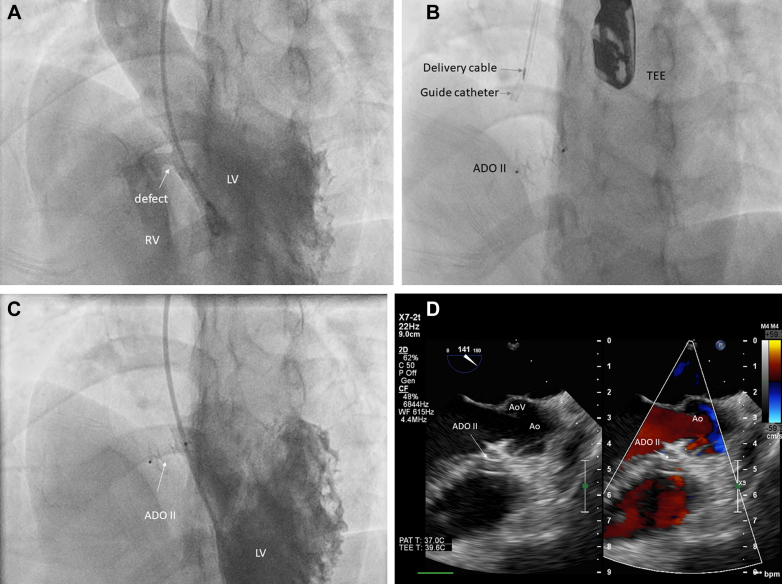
Fluoroscopy During Perimembranous Ventricular Septal Defect Closure Via Distal Radial Access Left ventriculography using a pigtail catheter at 45° left anterior oblique and 25° cranial views. (A) A left-to-right shunt was observed, and the size of the defect was 6 mm. (B) The ADO II device was released from the delivery cable. (C) Postprocedure ventriculography, with no leakage observed. (D) Transesophageal echocardiography (TEE) confirmed that there was no leakage at the defect. ADO II = Amplatzer Ductal Occluder II; Ao = aorta; AoV = aortic valve; LV = left ventricle; RV = right ventricle.
